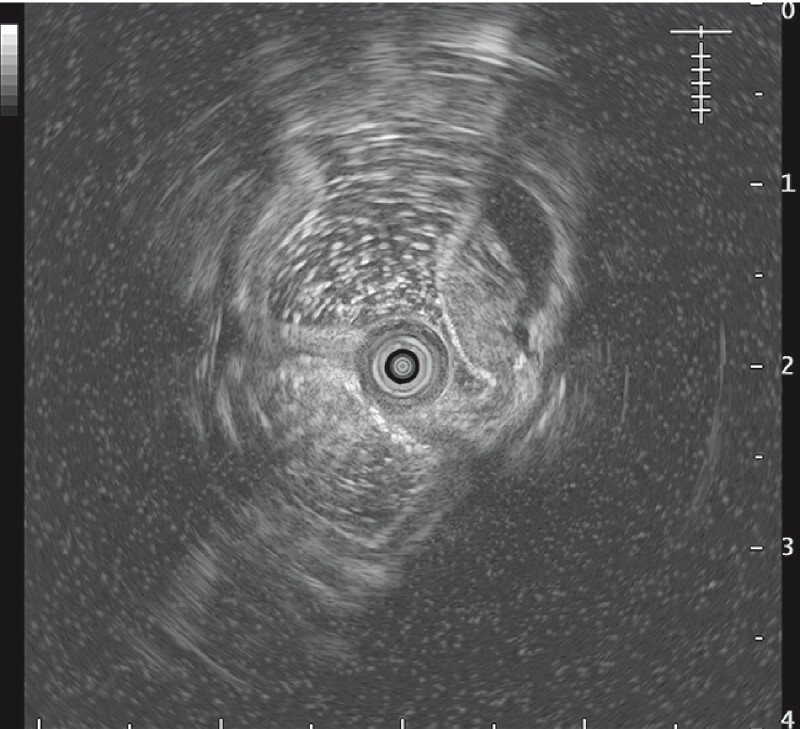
Endoscopic ultrasound confirmed the presence of a solid cystic lesion, which had a clear boundary and originated from the muscularis mucosae.
